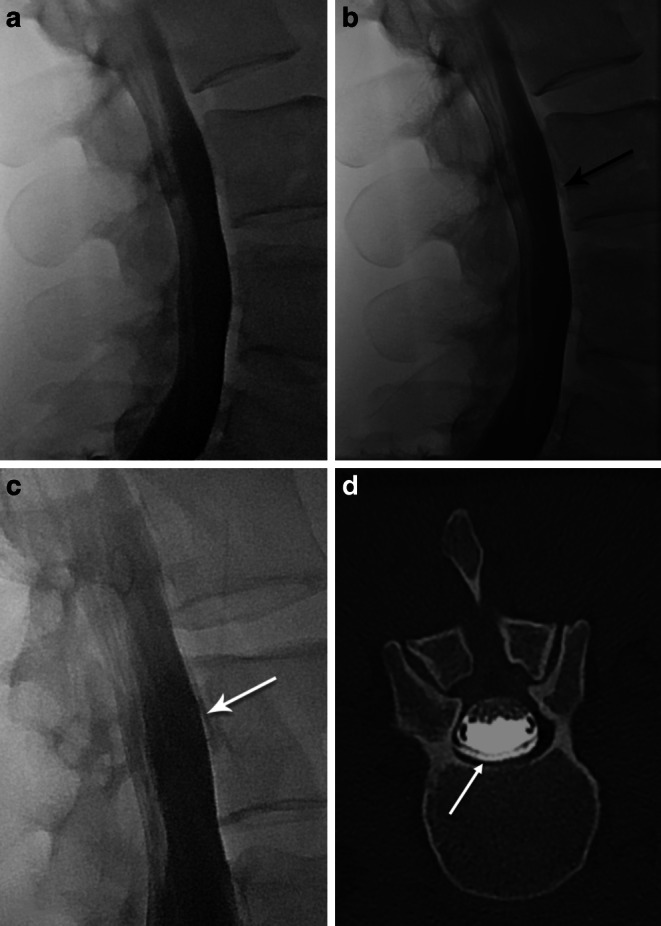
Middle-aged female with headache, neck pain, vertigo and nausea after spinal anesthesia. a–c Time course of dynamic myelography in sitting position with slow progression of contrast medium in the epidural space beginning at the level of vertebral body L3 b and progressing cranially c in the later phase. d Transversal postmyelography CT demonstrating contrast medium in the epidural space
